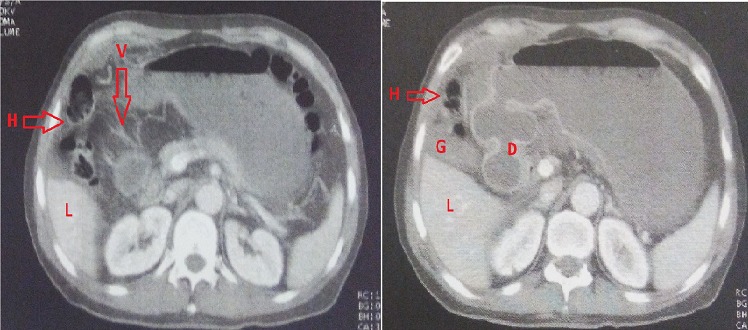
Abdominal CT scan, G= gallbladder, R= right Liver, D= duodenum, H = herniated loop, V= mesenteric vessels
